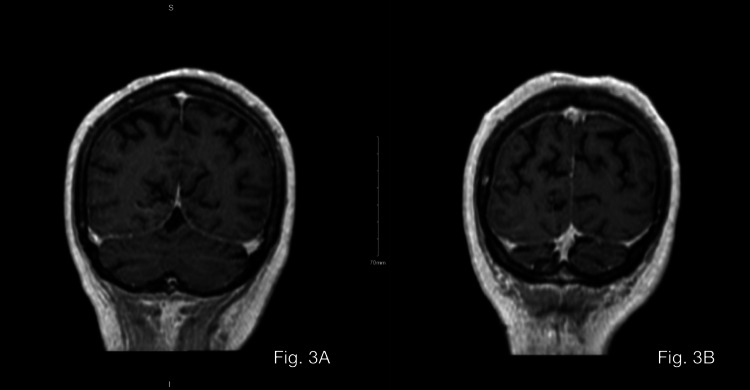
Brain T1-weighted MRI 3A–3B: Brain T1-weighted MRI (coronal view) demonstrating some cortical foci of bright T1 signal intensity with minimal blooming effects of gradient images. Also, there is diffuse cortical and leptomeningeal enhancement.
